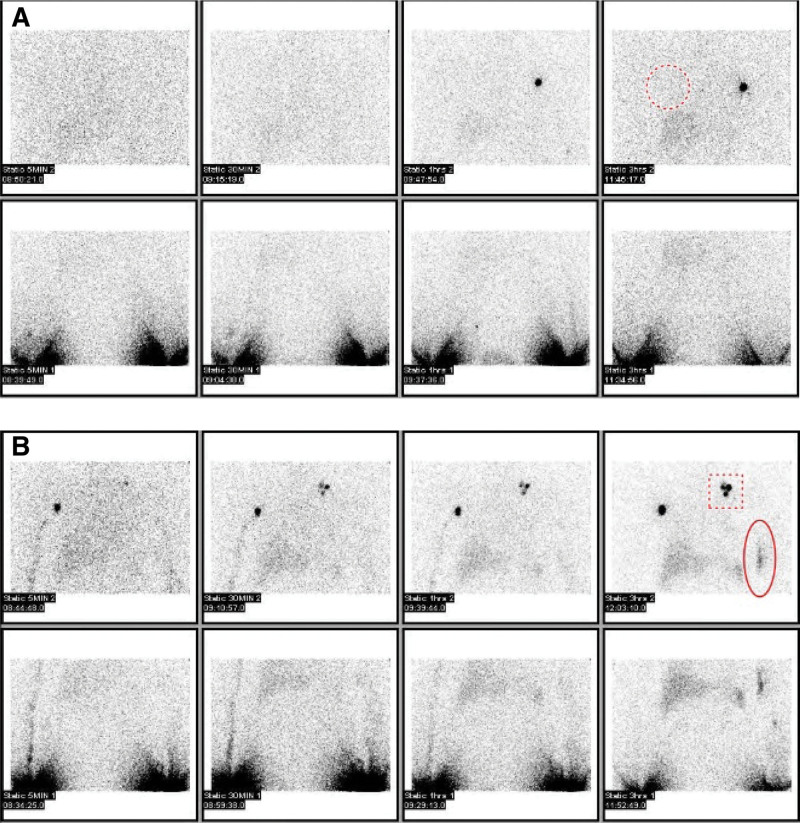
Abnormality at Lymphoscintigraphy. (A) Lymphoscintigraphy of a right breast cancer patient who underwent sentinel lymph node dissection. There are no axillary lymph node uptake on right side (dotted circle). (B) Lymphoscintigraphy of a left breast cancer patient who underwent left breast conserving surgery with sentinel lymph node dissection. There was dermal back flow (the dotted box) and a collateral pathway (oval) on the left upper limb.
